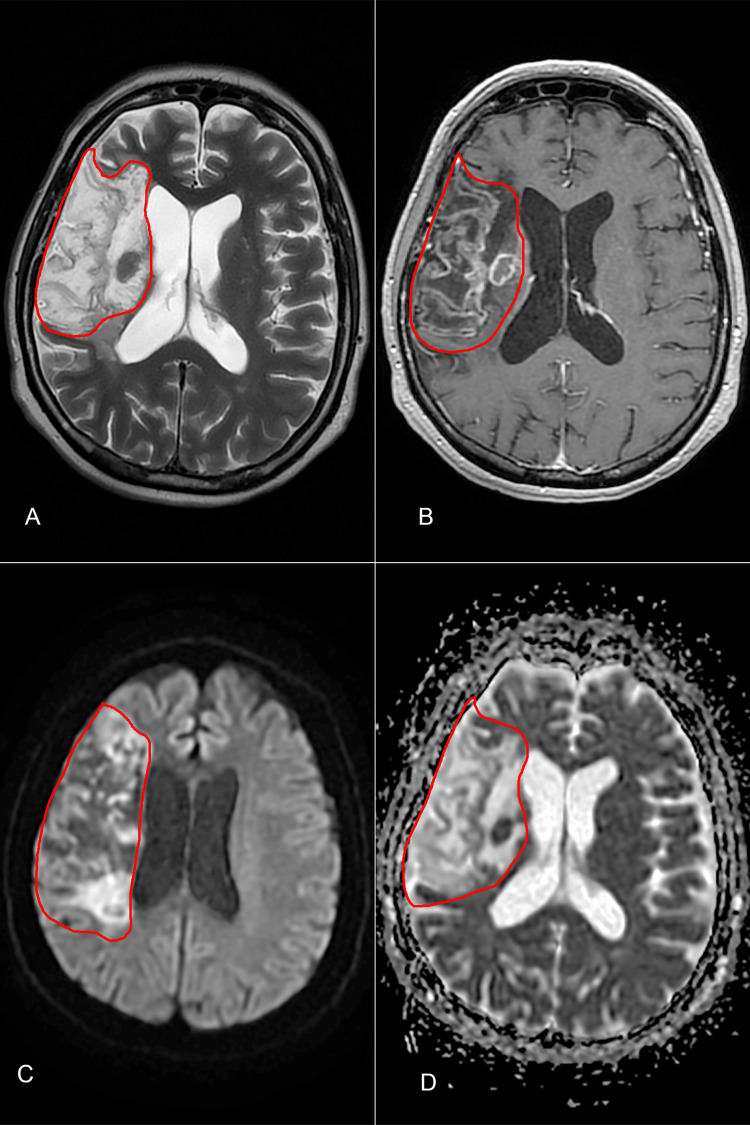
Cerebral MRI at the two-month follow-up depicting ischemic sequelae in the right frontoparietal region, with the area outlined in red. (A) T2 sequence; (B) T1 + C sequence; (C) diffusion-weighted imaging sequence; (D) apparent diffusion coefficient sequence.
